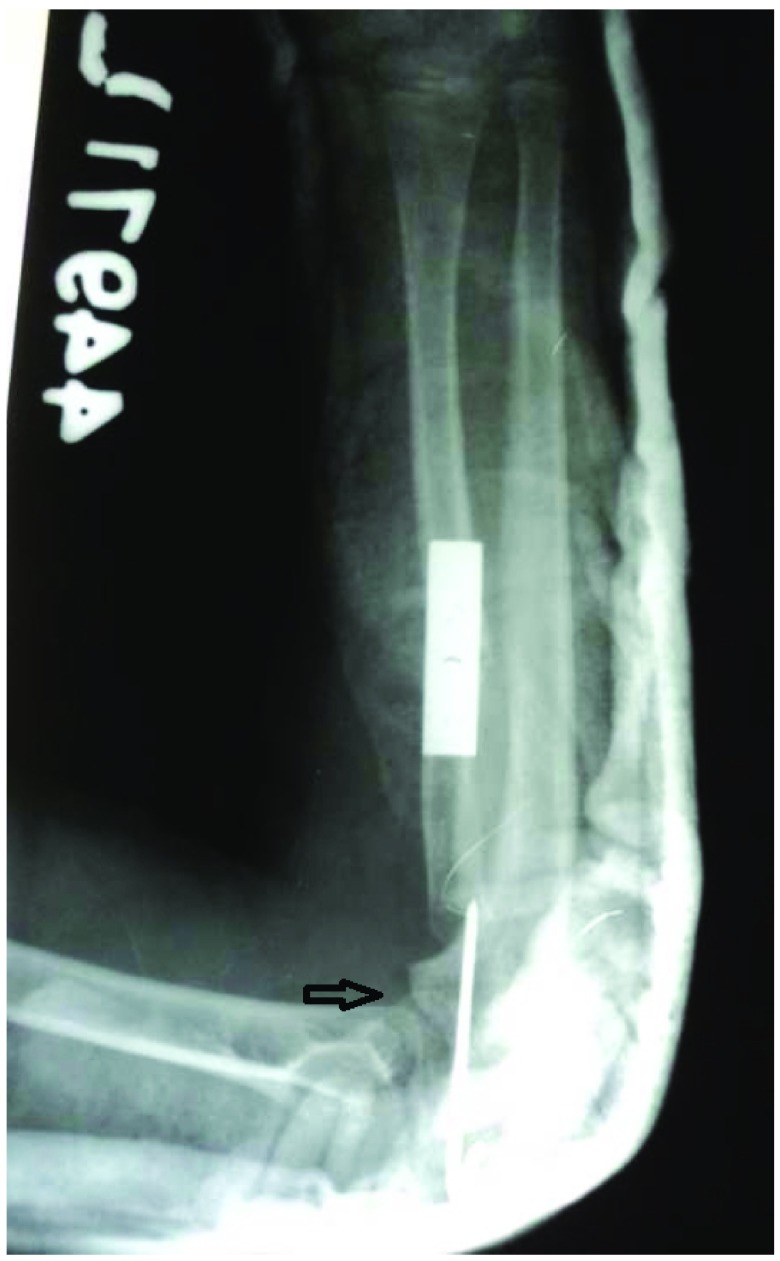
Immediate post-operative X-ray with radial shortening and fixation with plate and screws. The radial bow is corrected. The radial head is fixed with a transarticular pin.
